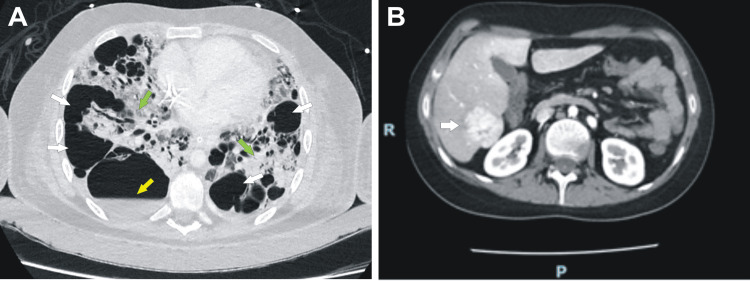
Chest and abdominal contrast-enhanced computed tomography. (A) Chest computed tomography showing progression of cystic lesions and bullae (white arrows), some of them with air-fluid levels (yellow arrow) coexisting with pulmonary infiltrates in the remaining parenchyma (green arrows). (B) Contrast-enhanced abdominal computed tomography showing a liver with a soft and regular contour and normal density. In segment VI, a 37 x 33 mm lesion was found (white arrow), showing an arterial-phase post-contrast enhancement.
